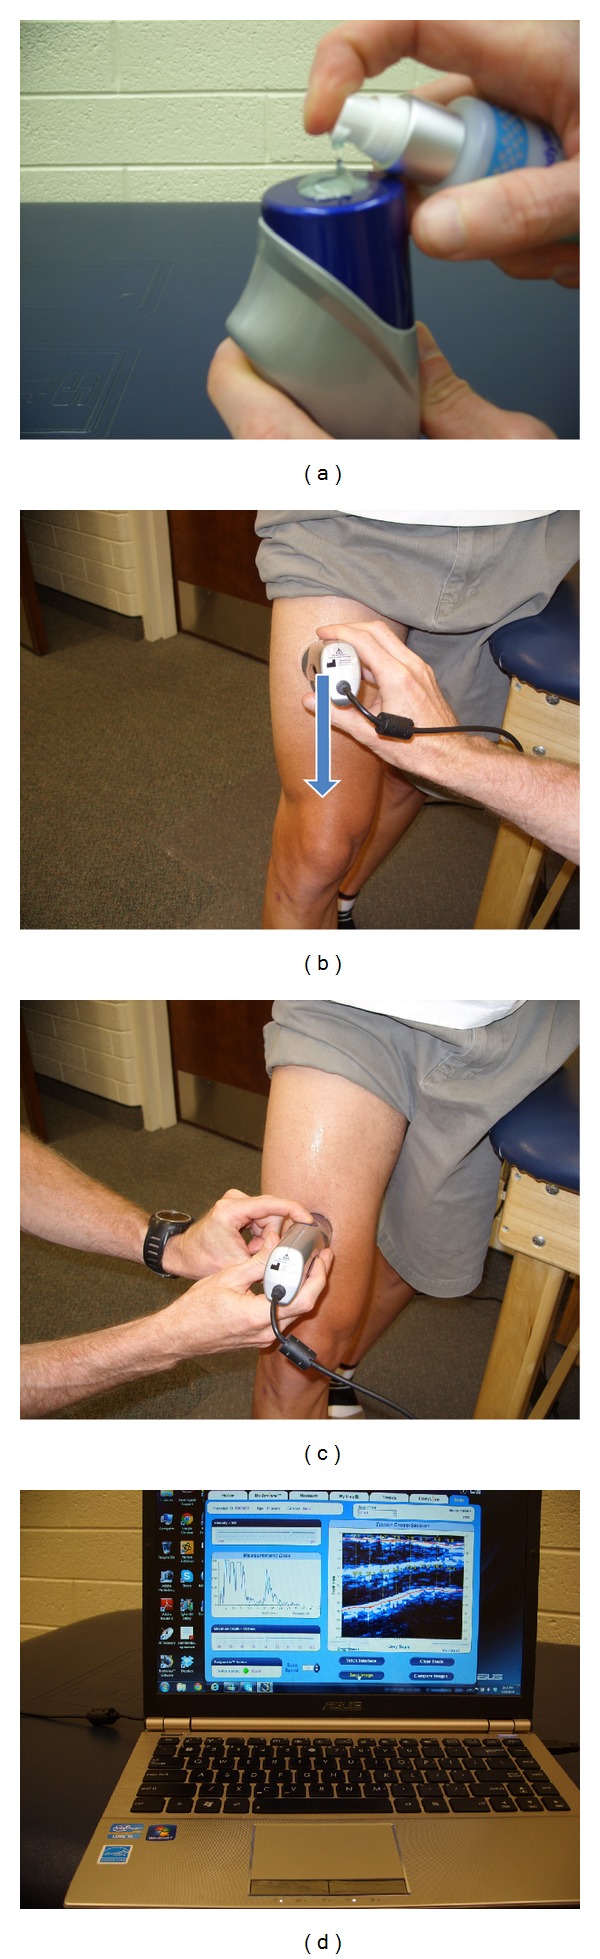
Measurement sequence for an ultrasound image scan of the thigh. (a) Gel applied to the ultrasound head for lubrication. (b) Beginning of scan. (c) End of scan. (d) Scanned image appears on screen and can be saved for future analysis.
